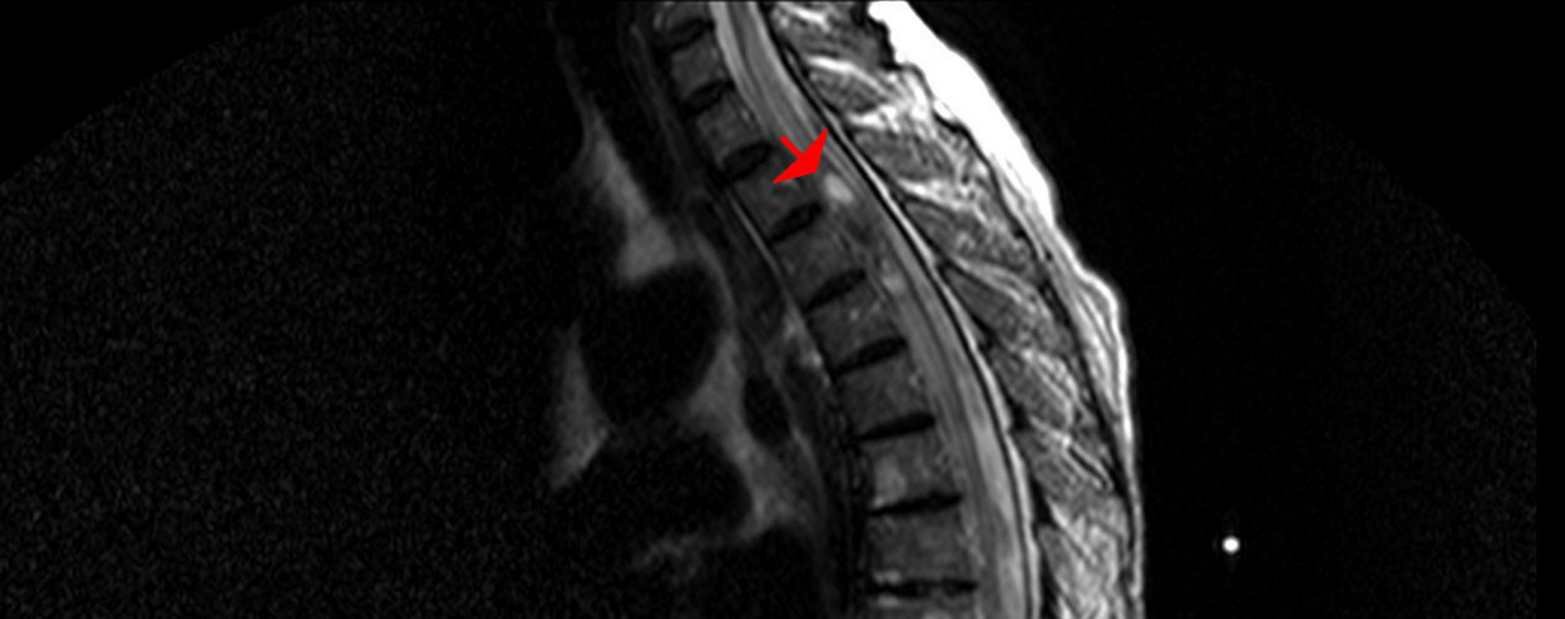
Sagittal T2 spinal MRI shows an intradural intramedullary tumor at T2, T3 level (hyposignal lesion with 2 hypersignal portion and proximal syrinx formation).
